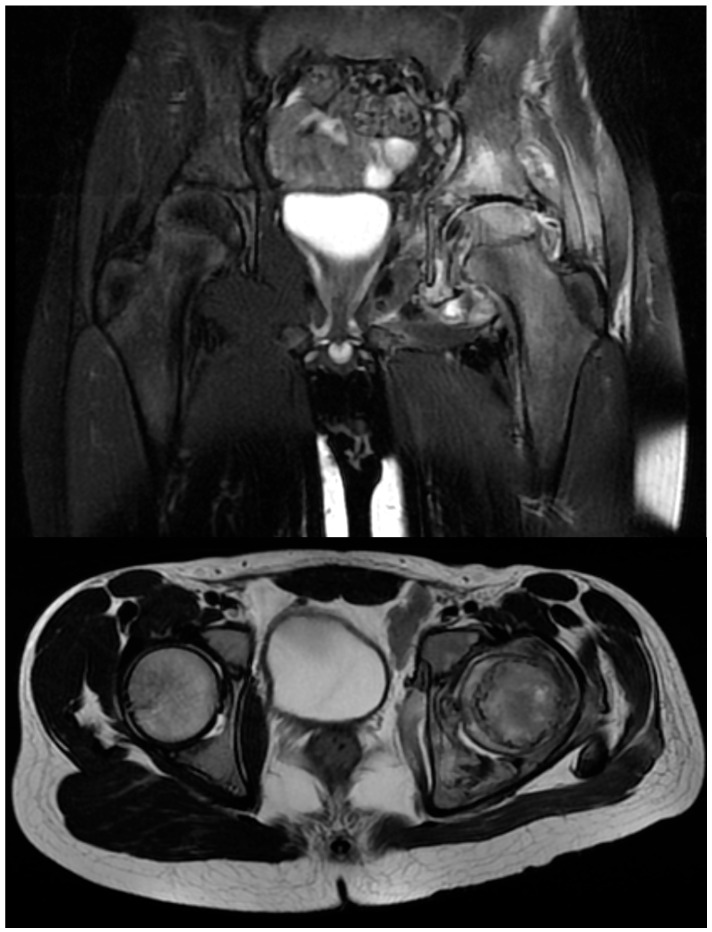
The pelvis MRI shows multiple osteoarticular changes, especially in the left ilium, ischium, and left femoral head, extending towards the neck. These areas display intense inhomogeneous T2 hypersignal and gadolinophilia, suggesting osteomyelitis. There is also an increase in the coxal bone diameter, loss of cortical continuity, uneven thinning of coxo-femoral cartilage, synovial hyperemia and hypertrophy, and increased septic synovial effusion. The infection extends into the gluteal regions and subcutaneous tissue, with significant periarticular soft tissue edema and notable left peri-iliac and peri-femoral lymphadenopathy.
